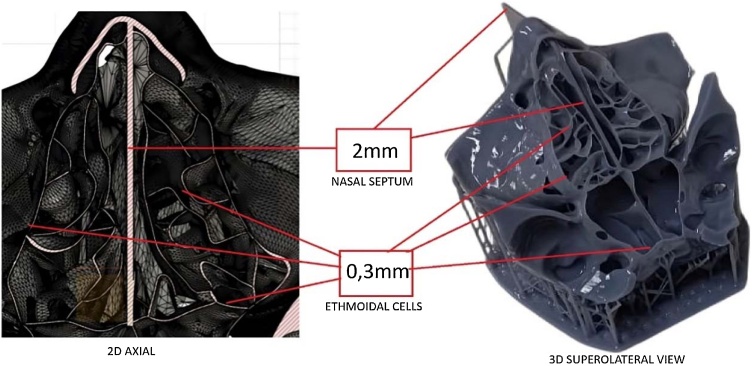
Two-dimensional and three-dimensional representations of the ethmoidal labyrinth based on computed tomographic data. Left: Axial computed tomographic highlighting the anterior and posterior ethmoidal cells. Right: superolateral view of the corresponding 3D-printed model in gray resin. All ethmoidal walls were printed with a uniform thickness of 0.3 mm, and a 2.0 mm nasal septum separates the two sides centrally.
